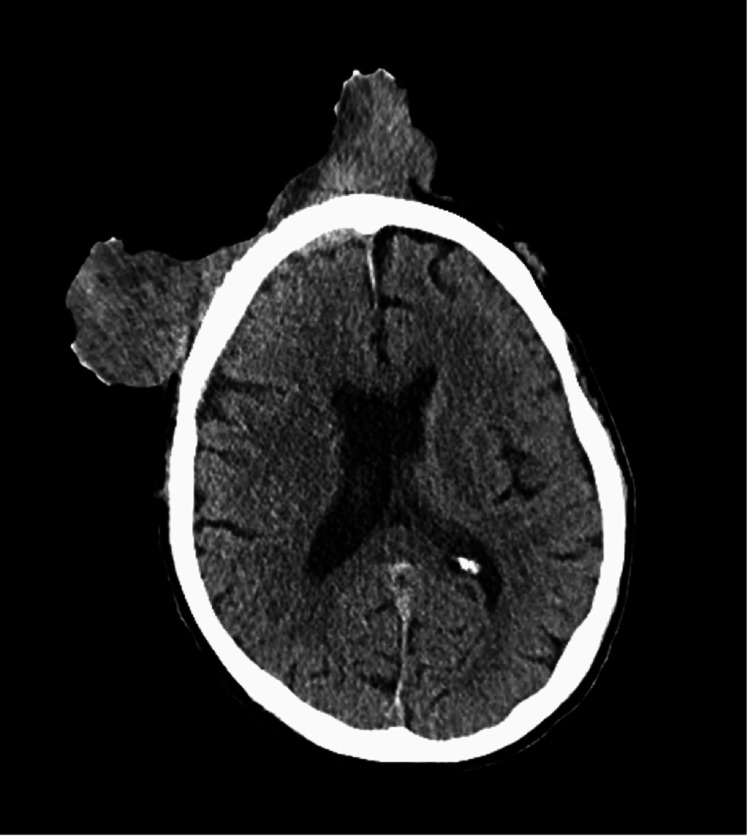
CT head with contrast showing an enhancing penetrating mass in the right frontal extra-axial space
